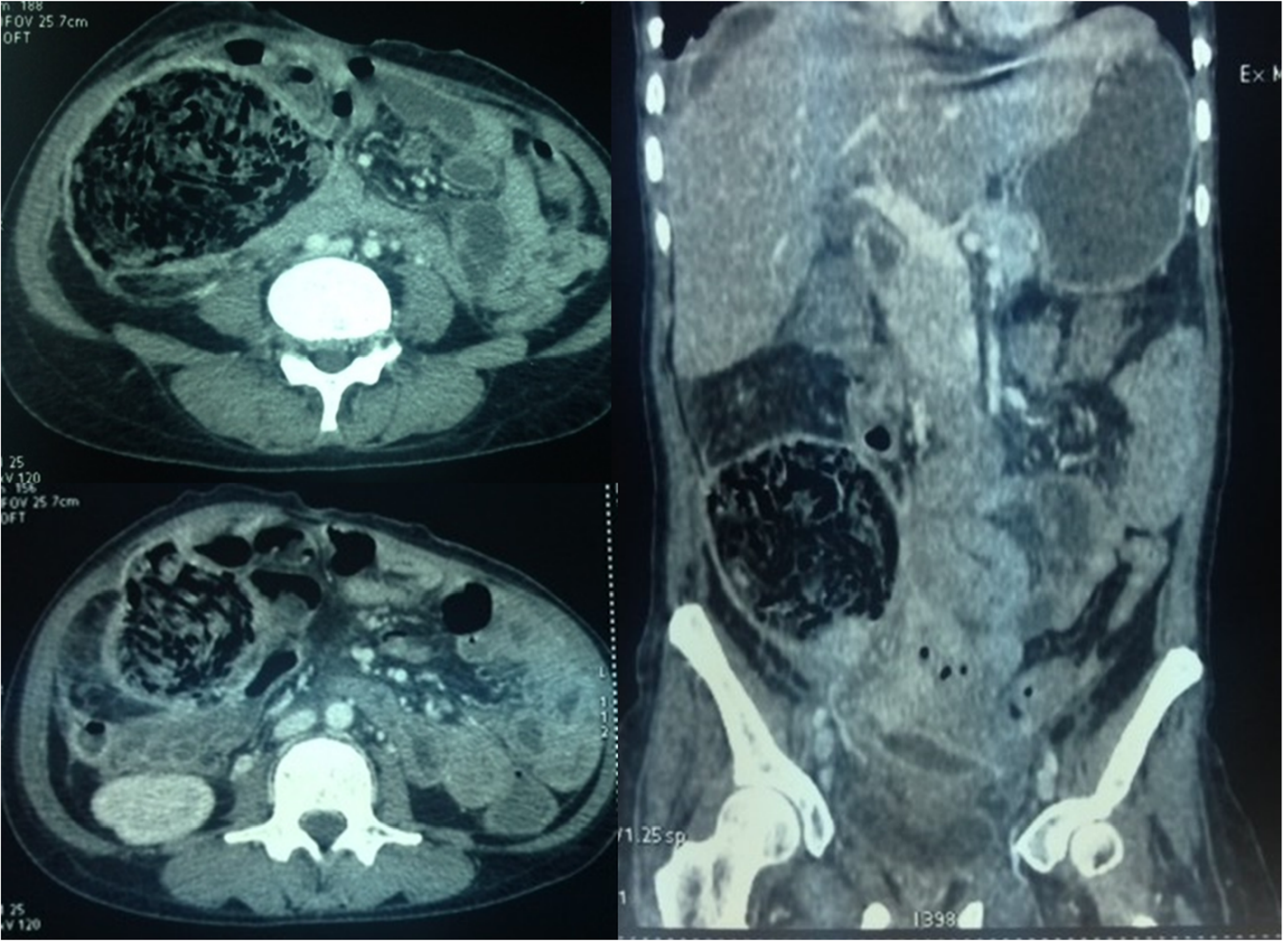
Axial (a and b) and coronal (c) abdominal CT scan images showingan heterogenous mass (gossypiboma) in the right flanc presenting a spongiform pattern
